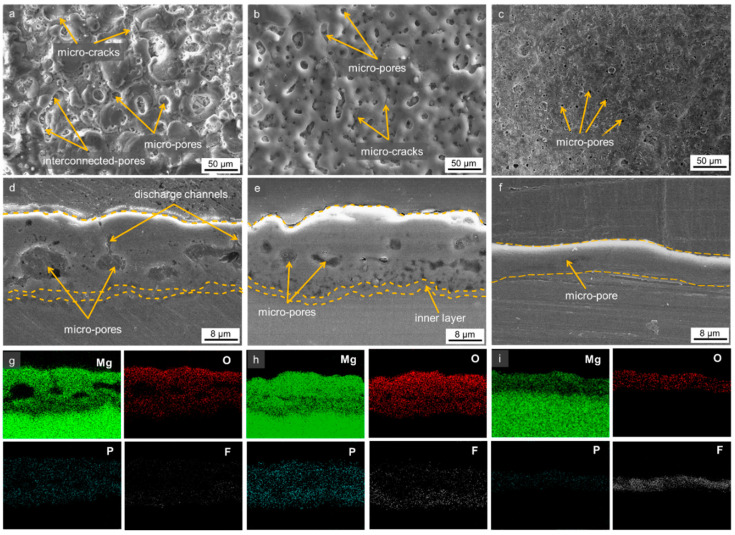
Surface morphologies and cross-sectional images of the oxide layer formed in three different ultrasonic frequencies of (a,d) 0, (b,e) 40, and (c,f) 135 kHz. EDS map area of Mg, O, P, and F elements on the surface of (g) U_f_0, (h) U_f_40, and (i) U_f_135.
